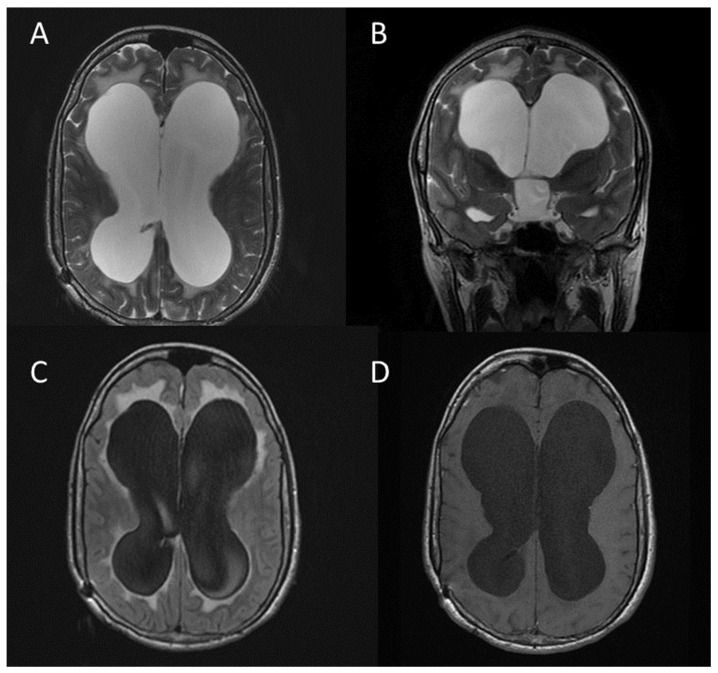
Hydrocephalus in a patient with NF1. Axial T2-weighted FRFSE (A); coronal T2-weighted FRFSE (B); axial T2 FLAIR (C); axial T1-weighted FSE (D) [11].
